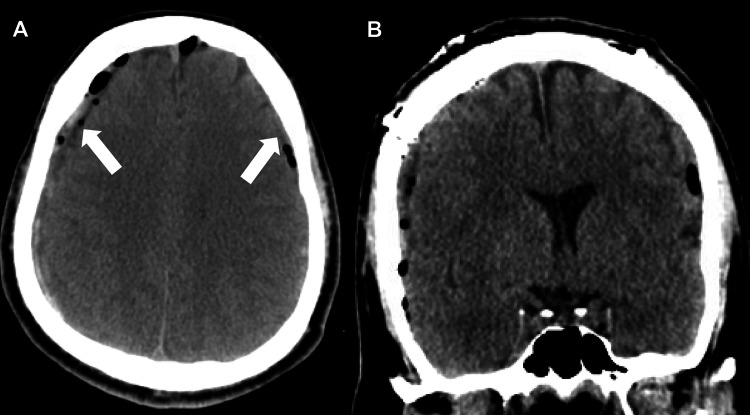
Immediate postoperative non-contrast CT of the head after right-side craniotomy and left-side burr holes for bilateral subdural hematoma evacuation. (A) Axial section and (B) coronal section showing small residual subdural hematoma bilaterally (arrows).
